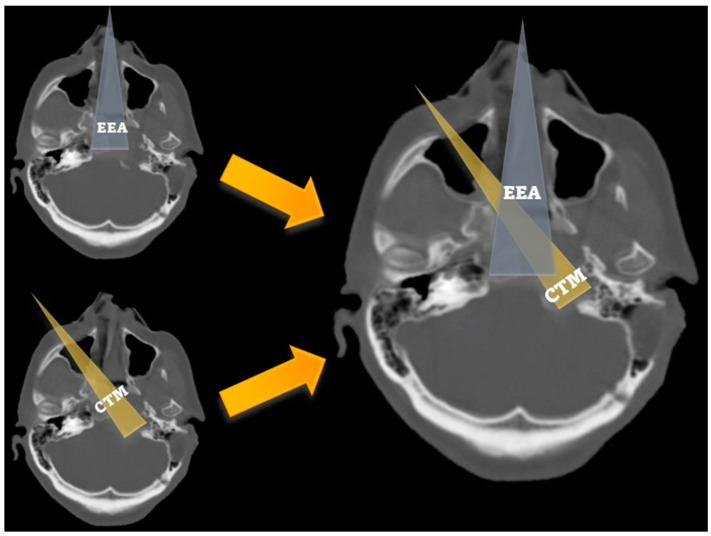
Bone CT scan comparing the trajectories and the area of exposure related to the endoscopic endonasal (grey) and the contralateral transmaxillary (yellow) approaches, showing that the endonansal corridor provides a good exposure of the medial clivus, while contralateral transmaxillary corridos allow reaching the lateral clivus and the petroclival synchondrosis.
